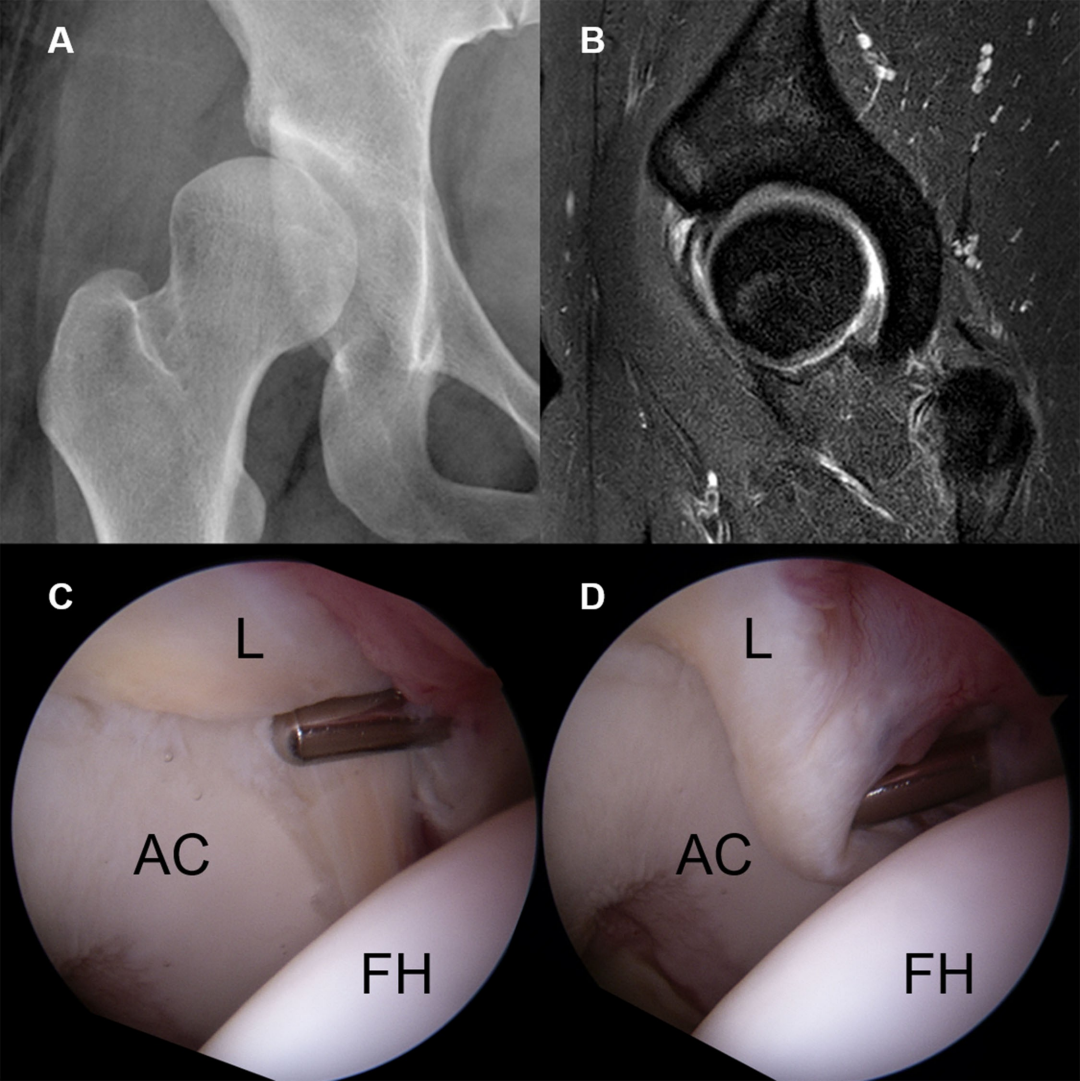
A grade 3 labral injury in a 26-year-old woman. (A) Simple X-ray showing a definite dysplastic acetabular structure. (B) Sagittal hip MRI showing increased intrasubstance signal intensity and a contrast material-filled paralabral cyst at the chondrolabral junction. (C, D) Arthroscopy showing an unstable labrum between the chondrolabral junction (C) and the capsulolabral recess (D), without labral displacement. (L, labrum; FH, femoral head; AC, acetabular cartilage).
